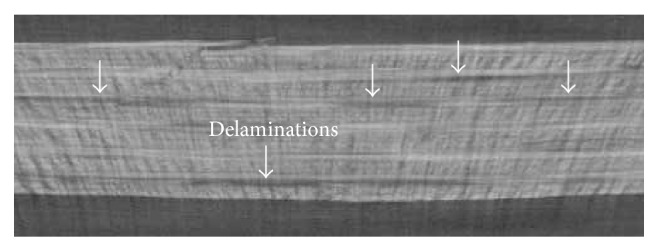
X-ray microtomography image of the impacted Twintex laminate (sample delamination zones are shown with arrows).
